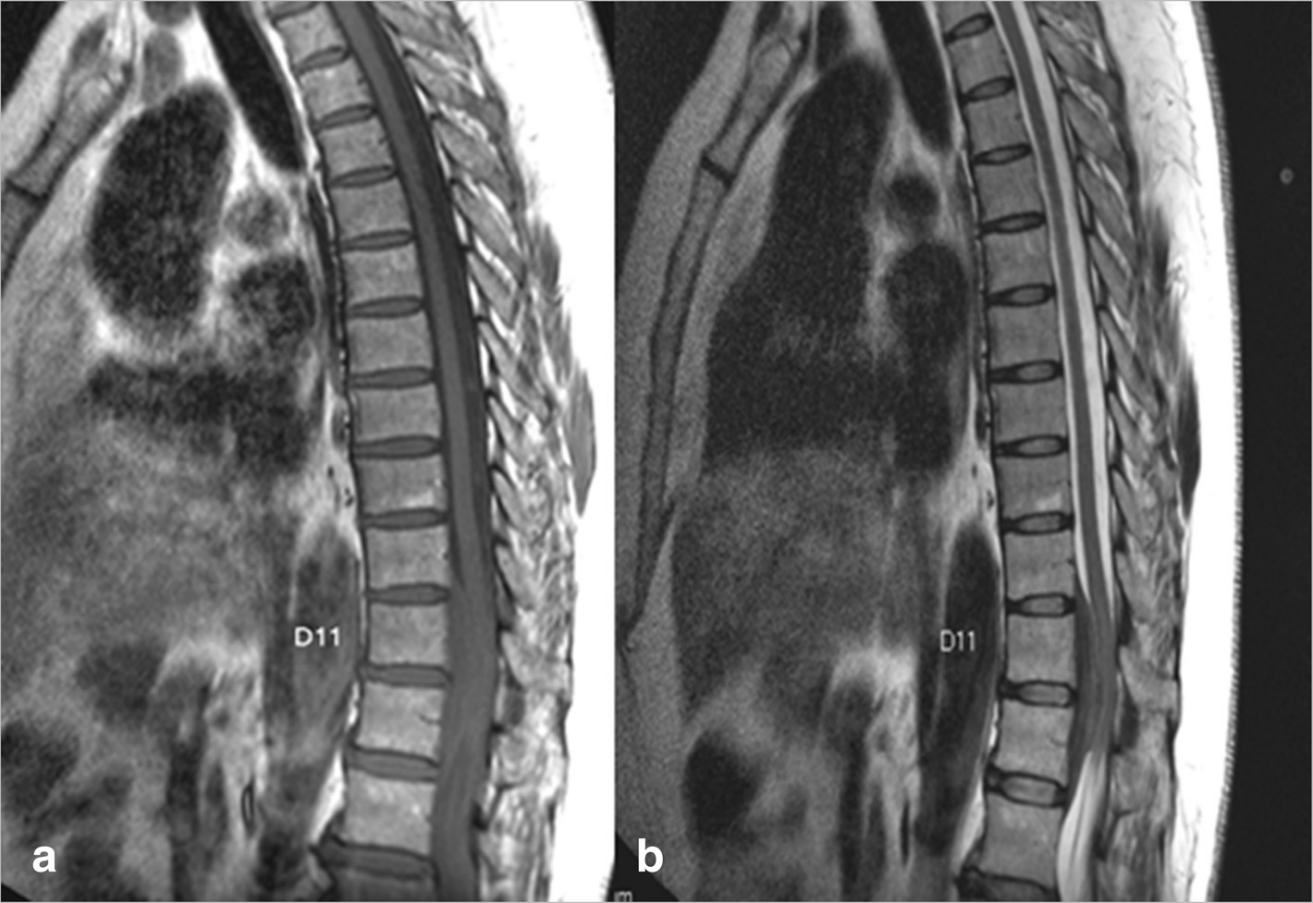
Gadolinium-enhanced magnetic resonance images showing an epidural mass at the D10–D12 level with cord compression and intense homogeneous contrast enhancement in a T1 series. a T1 sagittal view. b T2 sagittal view
